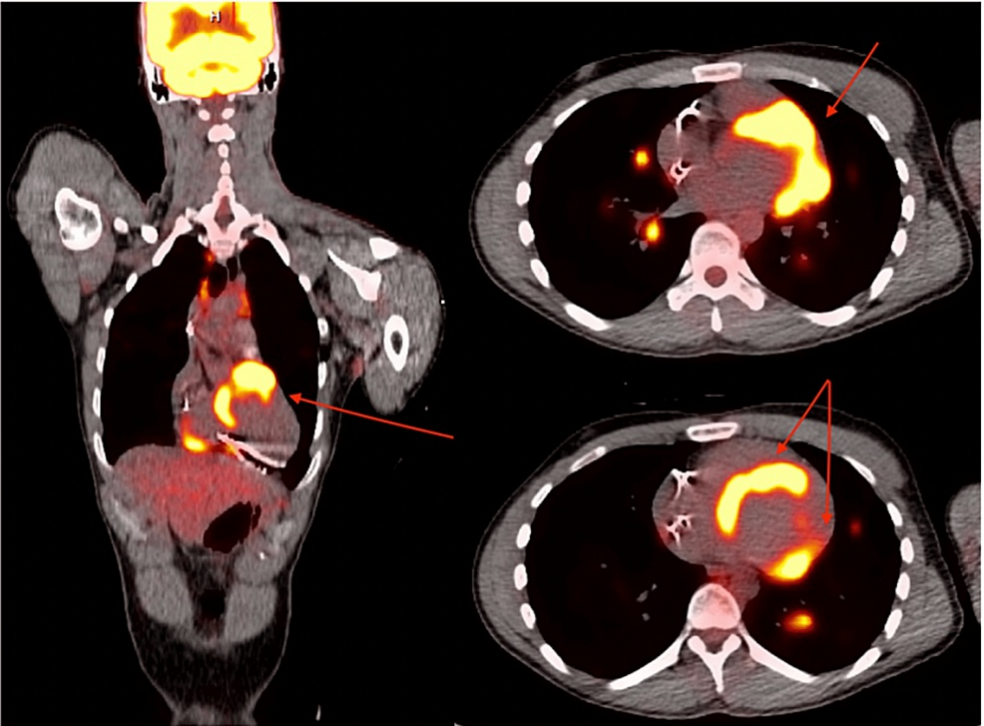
Cardiac PET scan indicating FDG uptake in the septal basal lateral, inferior, and anterior walls with additional uptake in the RV basal wall. PET: positron emission tomography; FDG: fluorodeoxyglucose; RV: right ventricle
